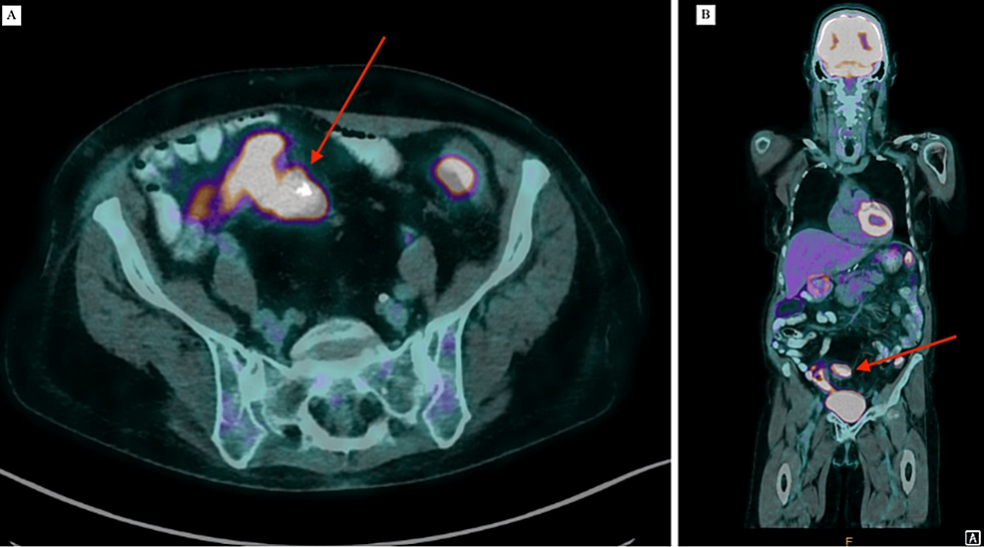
FDG-PET scan 2023, axial (A) and coronal (B) views demonstrating a 30x37mm avid mass within the sigmoid mesentery. FDG-PET: Fluorodeoxyglucose Positron Emission Tomography
